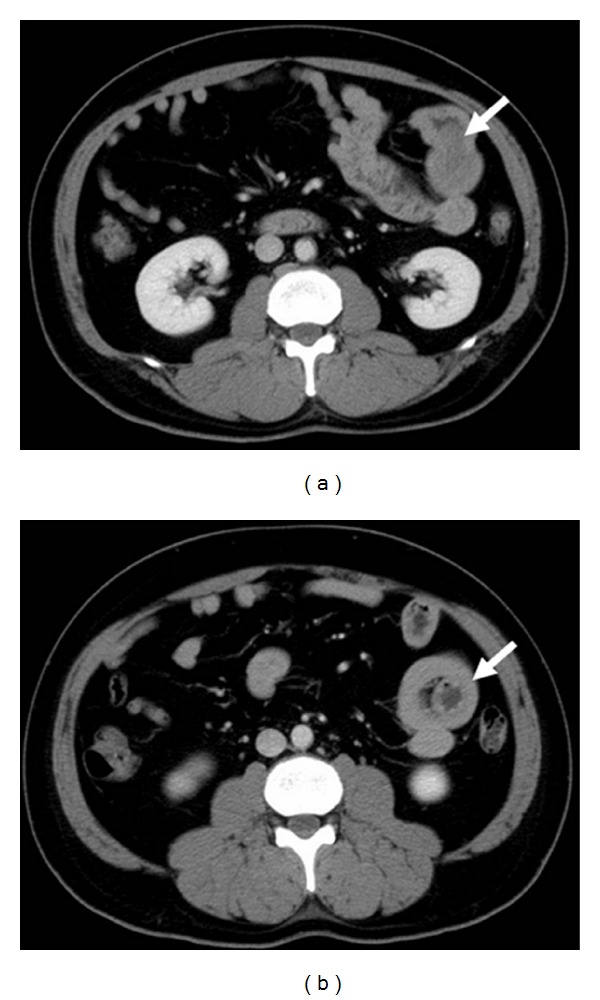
CT findings. (a) Abdominal CT showed the small bowel tumor (arrow). (b) Invagination of a part of the small bowel (arrow).
